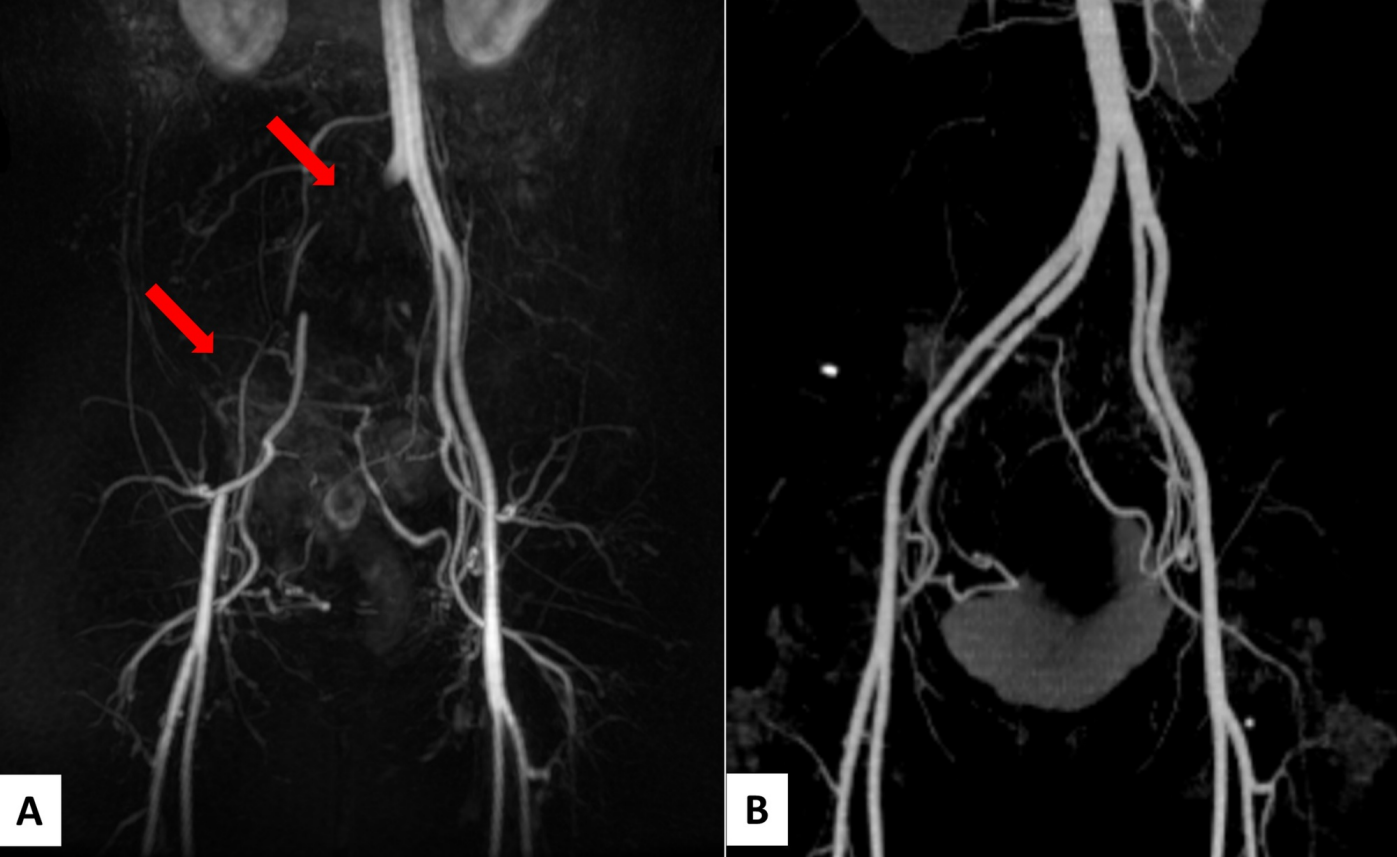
Time-resolved contrast-enhanced magnetic resonance angiography (MRA) images and CT angiography one month later. (A) Maximum intensity projection (MIP) of time-resolved contrast-enhanced MRA images show lack of enhancement of right common iliac artery and proximal external iliac artery (arrows). Distal arterial branches are visualized via internal iliac circulation. (B) MIP of CT angiography shows normal opacification of bilateral iliac and femoral arteries, and no vascular wall abnormality is recognized.
